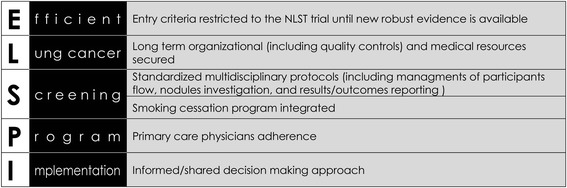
Proposed outline for implementing an efficient, low-dose computed tomography lung cancer screening program
